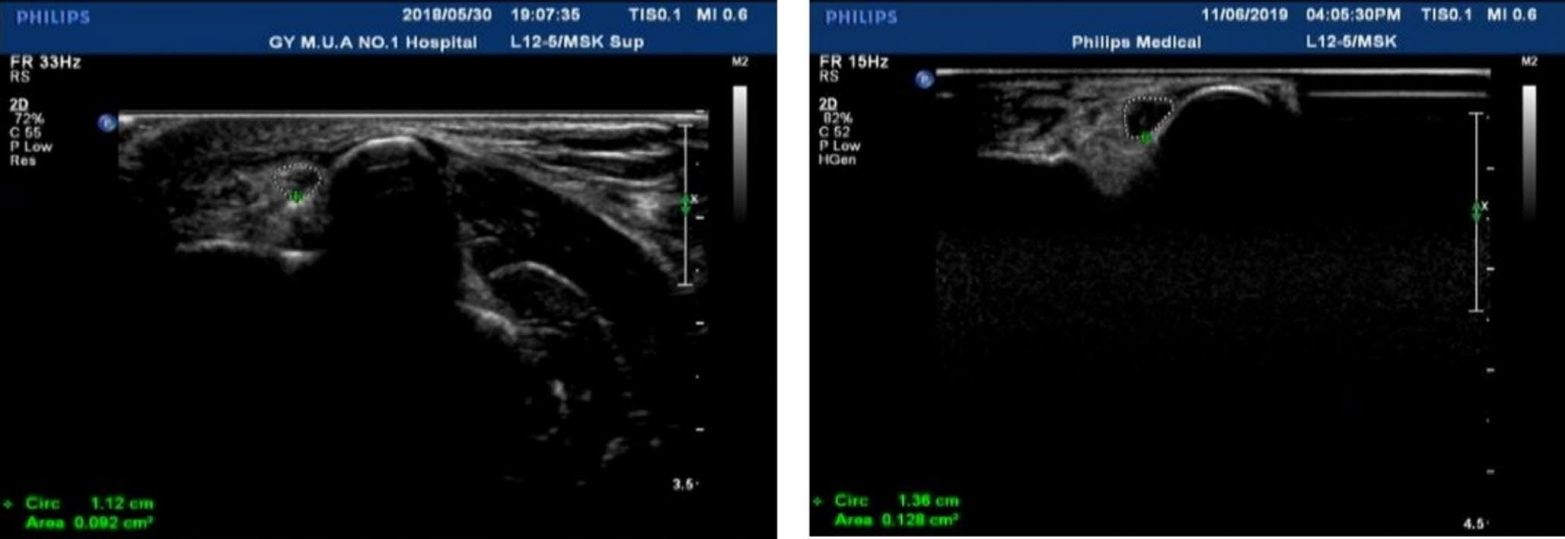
Cross sectional area of ulnar nerve in cubital tunnel under high frequency ultrasound.The cross-sectional area of normal ulnar nerve in cubital tunnel was 0.092cm^2^ (A); In the cross section of ulnar nerve in cubital tunnel of patients with diabetic peripheral neuropathy under high-frequency ultrasound, the internal nerve bundle structure was blurred, no hyperechoic nerve adventitia was found, and the cross-sectional area was 0. 128 cm^2^ (B)
